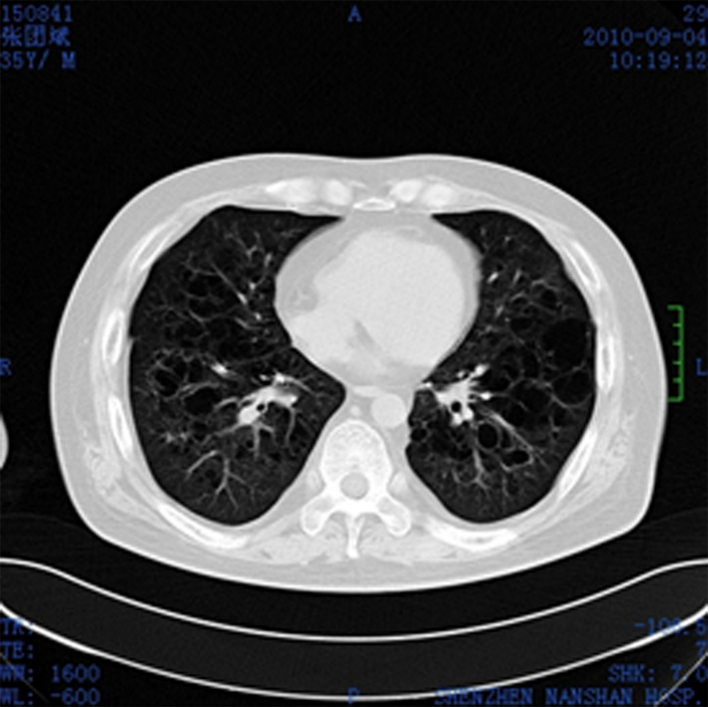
Brest CT plain scan Higher penetrance of lung fields, extensive unequal-sized flake or cystic bright shade, and incrassate shade of interlobular septum.
